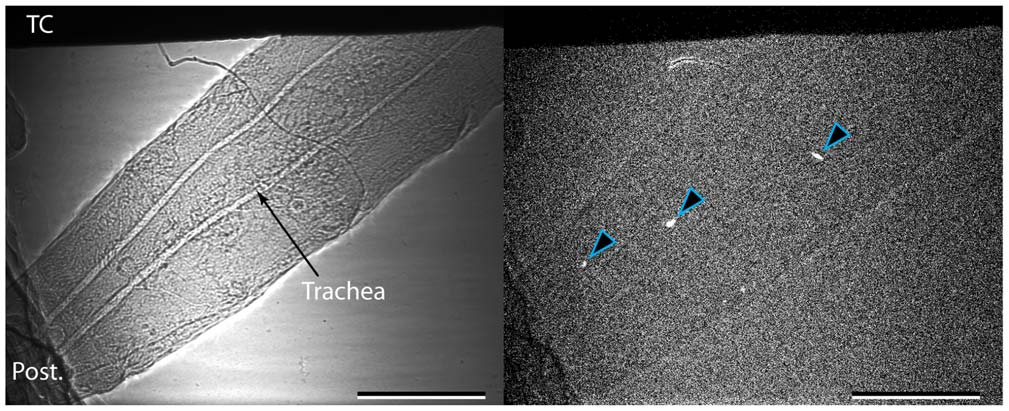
Isolated freezing events during freezing. Example of isolated freezing events visible in some ice formation sequences, in this case in a diapausing *C. amoena* larva with an SCP of −8.1°C. Left hand image is the phase-contrast x-ray image, right hand is a subtraction of two consecutive images collected c. 0.3 s apart, and white patches (indicated with arrows) are freezing events that appear to be an entire compartment of water freezing. These events are also visible in movies S1, S2, S3 and S4. TC indicates the thermocouple, ‘Post.’ the posterior end, which in this case is in contact with the wall of the chilling chamber. Scale bar is 0.5 mm.
